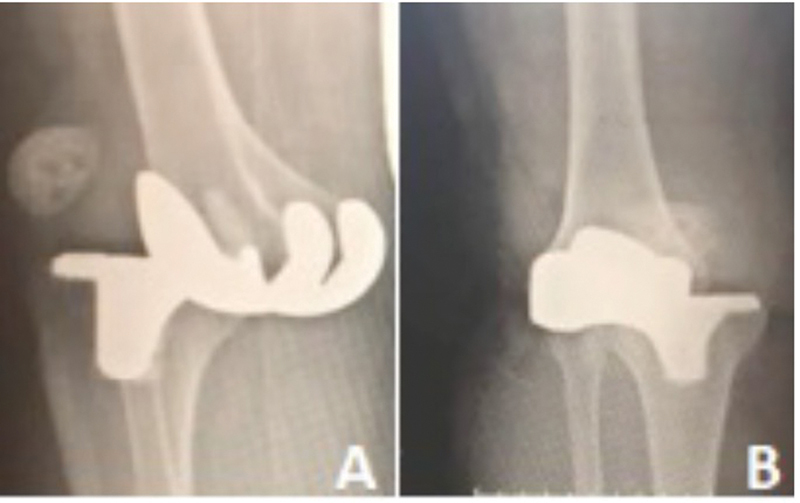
Anteroposterior pre reduction radiographic assessment (
